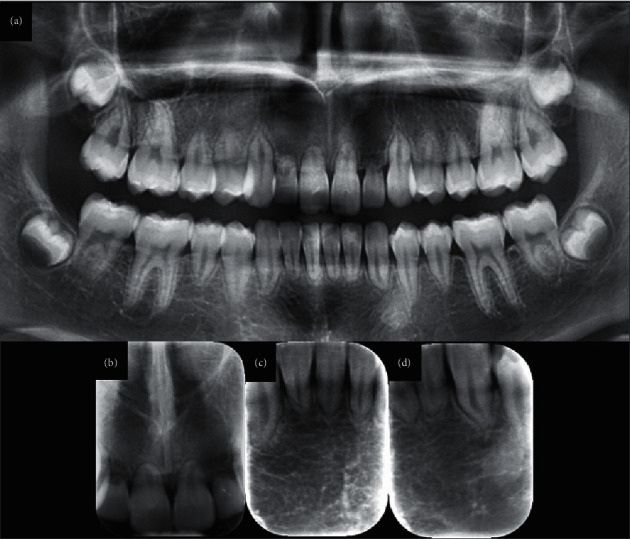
Initial radiographic examination of SRA (age of patient: 11 years and 9 months). (a) Panoramic radiography. (b) Periapical radiograph of the maxillary incisors. (c) and (d) Periapical radiographs of the lower incisors. Notice the abnormally short roots with round apices of all teeth except first molars. Mandibular first premolars also have short roots, but their root to crown ratio is bigger than 1 : 1. Maxillary lateral incisors present intense root resorption and apical radiolucency.
